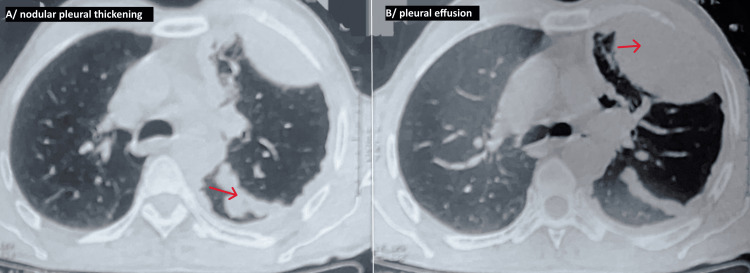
A chest CT scan with parenchymal window revealed nodular pleural thickening on the left side, with a moderate left pleural effusion, and emphysematous lungs. A: The red arrow marks the nodular pleural thickening. B: The red arrow marks the moderate pleural effusion.
